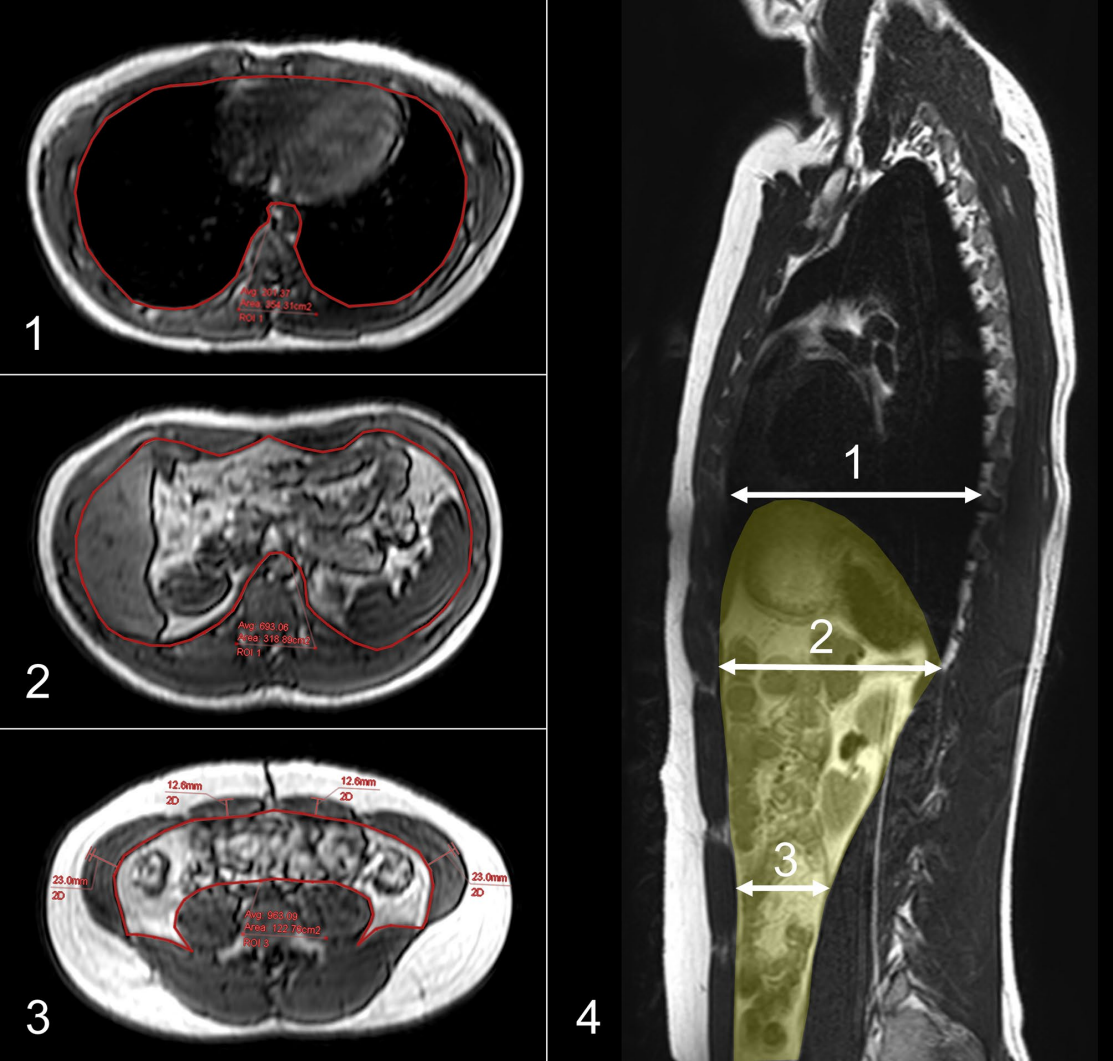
Measurements of the cross-sectional area of the abdominal cavity at three levels. 1 Axial MR image at the upper liver level showing measurements of cross-sectional area. 2 Axial MR image at the lower lung level showing measurements of cross-sectional area. 3 Axial MR image at the umbilical level showing measurements of cross-sectional area and thickness of the abdominal muscles. 4 Sagittal MR image at the left kidney level showing abdominal cavity, which is marked in yellow. (1) indicates the upper liver level, (2) indicates the lower lung level, and (3) indicates the umbilical level.
